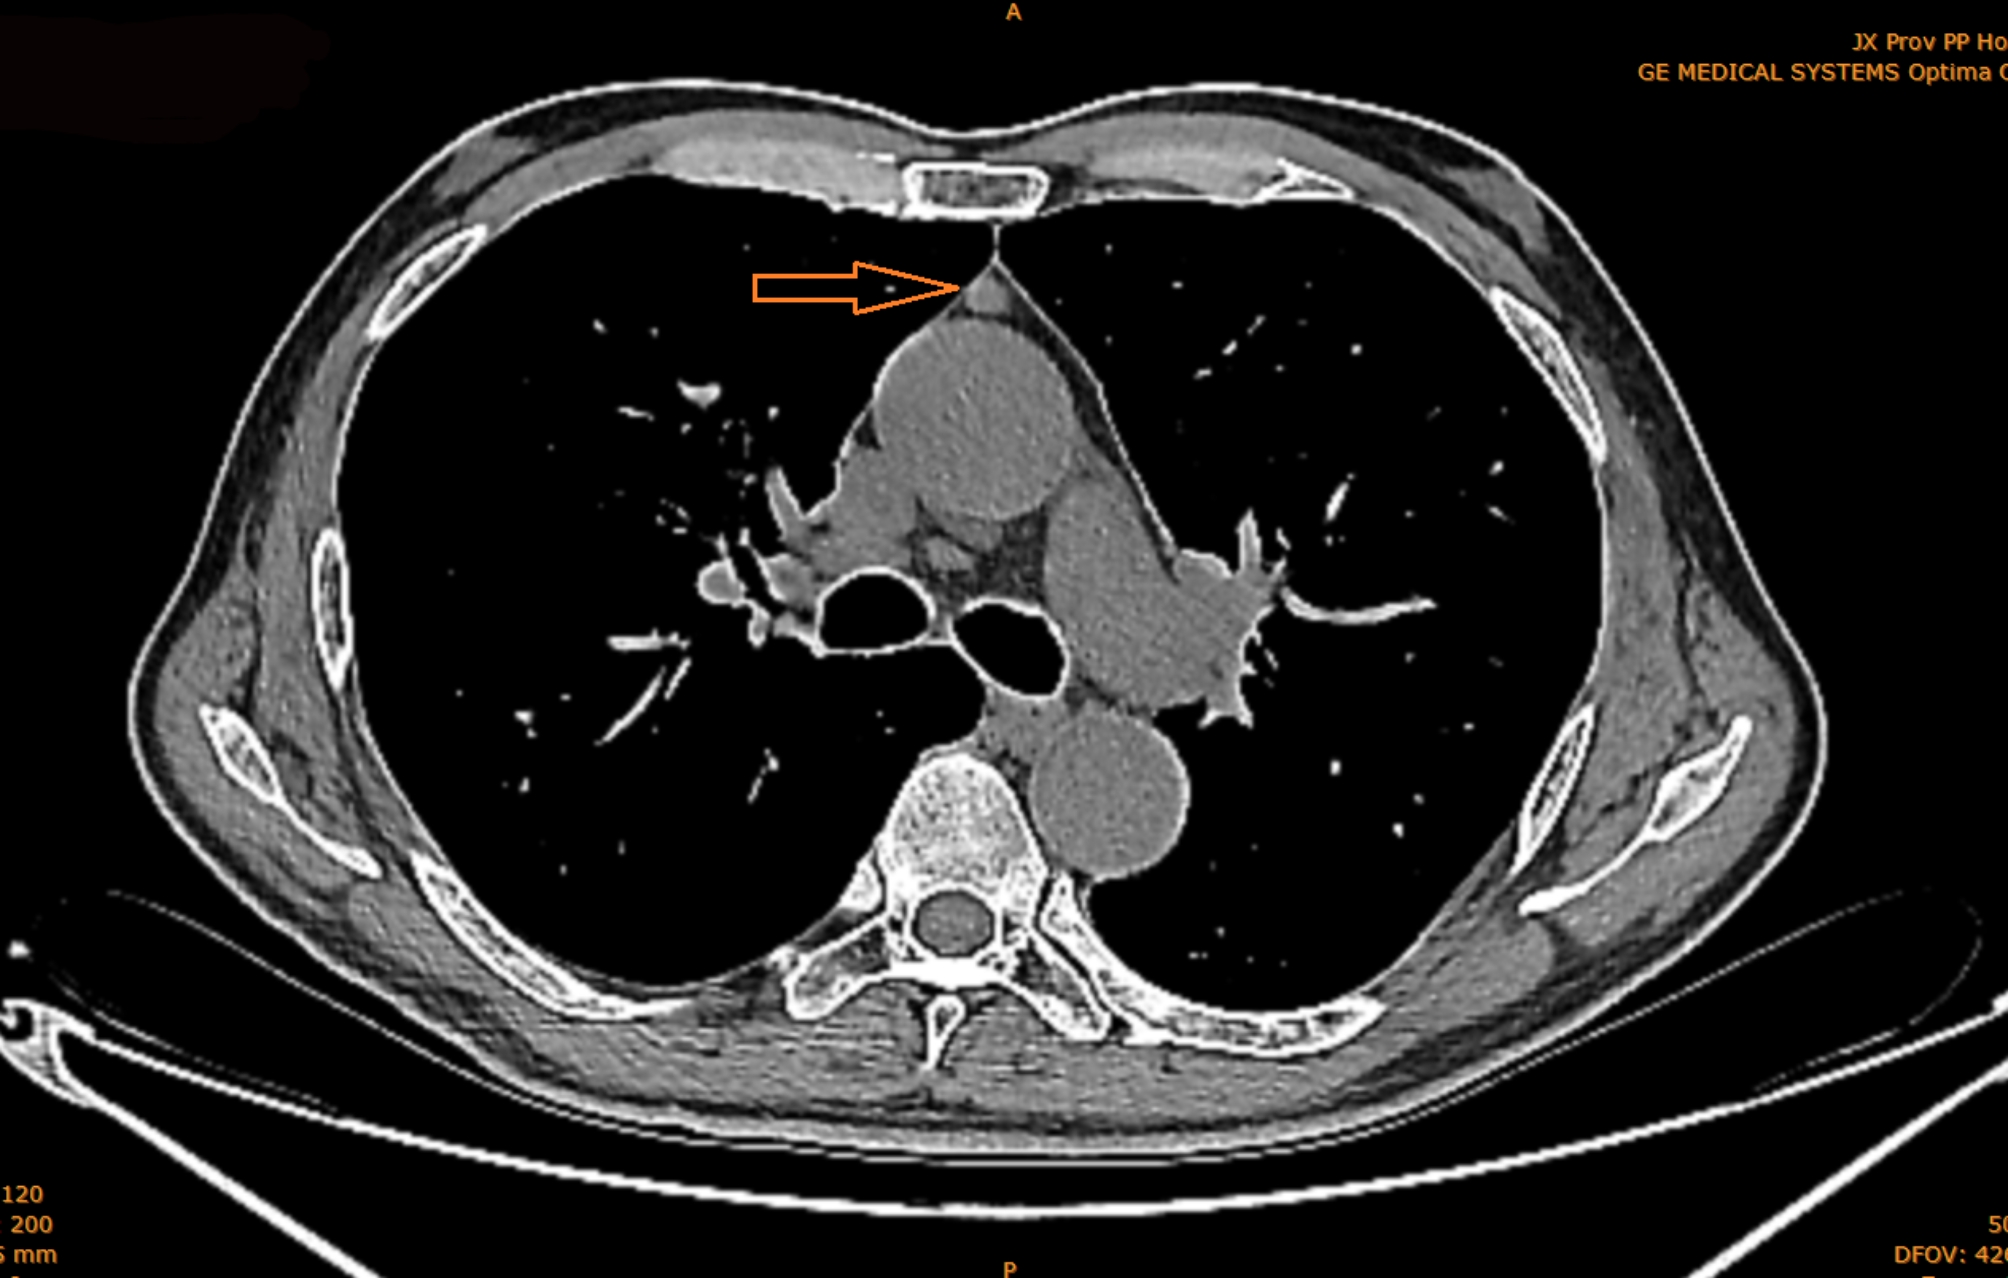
Computed tomography scan, showing an isolated and well circumscribed soft tissue density (1.2 × 1.0 cm) in the anterior mediastinum
